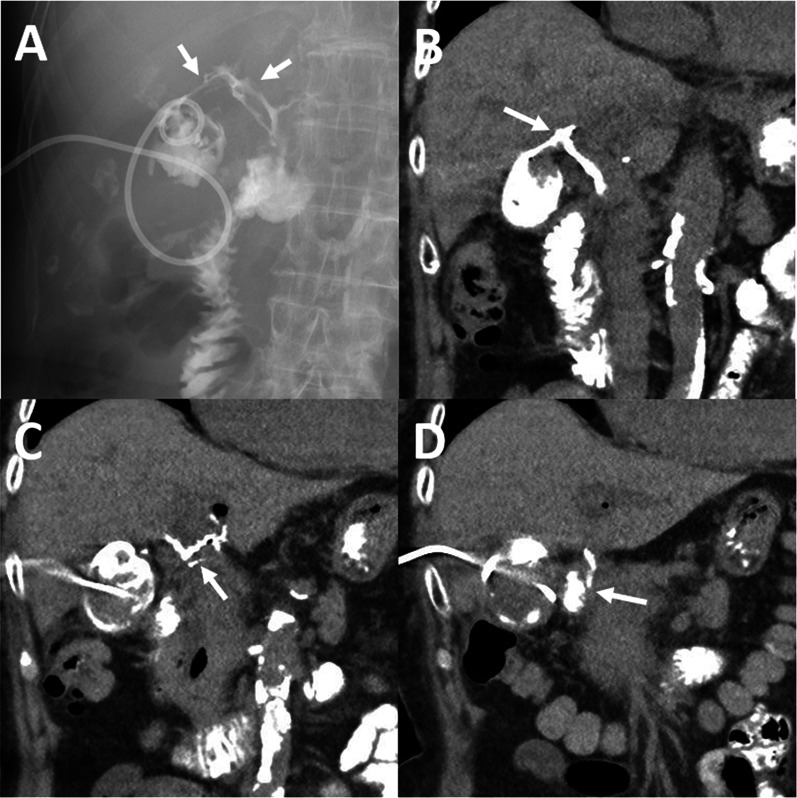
Radiographic contrast examinations. A The contrast medium from the PTGBD tube flowed out to the duodenum through the hepatoduodenal ligament (arrows). The cystic duct and extrahepatic bile duct were not contrasted. B, C CT showed that the contrast medium spread into the hepatoduodenal ligament (arrows). D CT showed that the contrast medium in the hepatoduodenal ligament connected to the duodenum (arrow)
